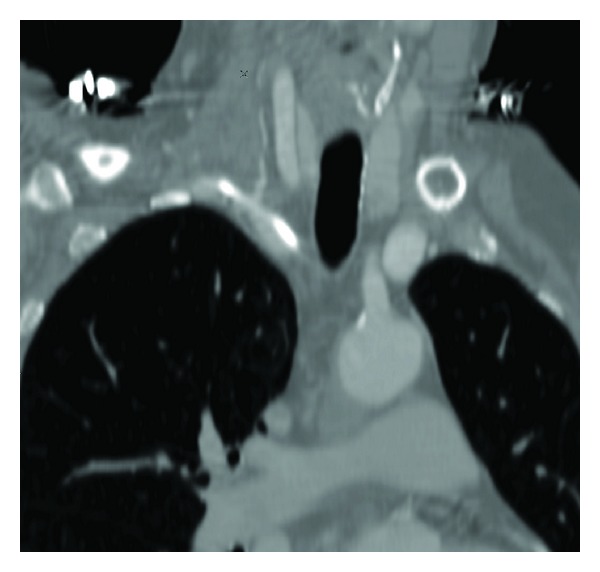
Oblique reformat of CT angiogram showing the entry site of the catheter into the artery and location of the origin of the vertebral artery.
